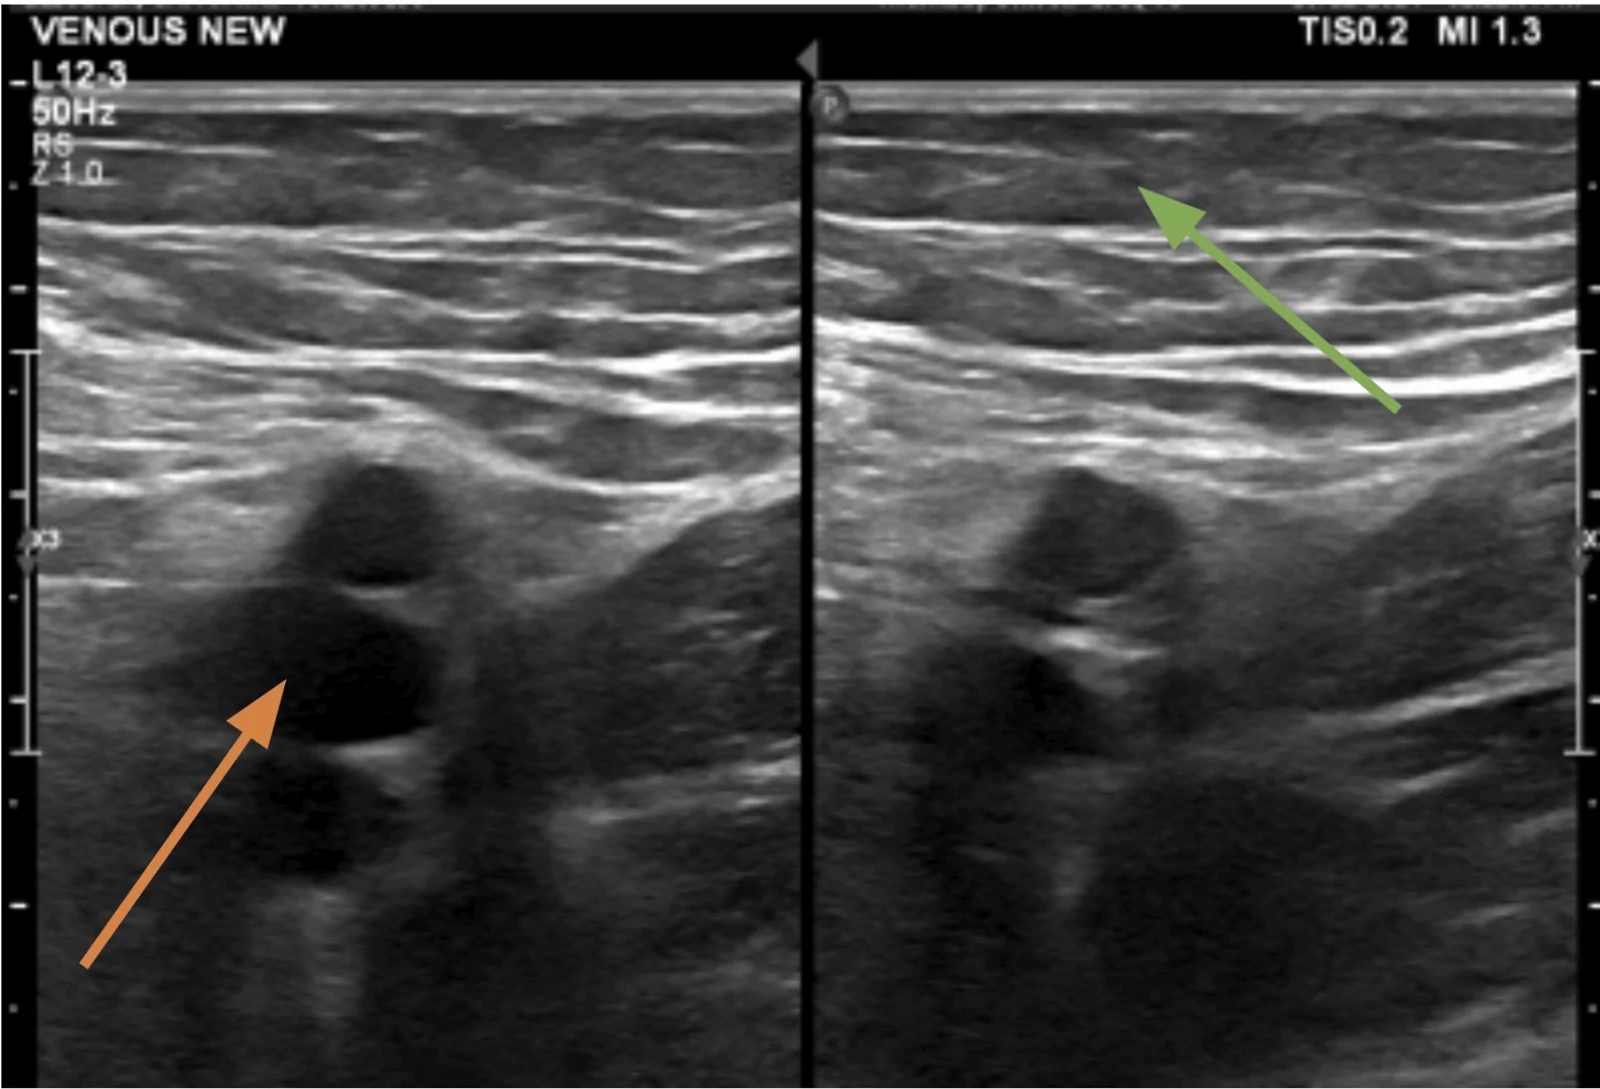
Left lower limb ultrasound The orange arrow points to the fluid streaking at the ankle region, with fluid collection at the tibialis anterior tendon. The green arrow denotes the subcutaneous soft-tissue edema along the left lower leg
